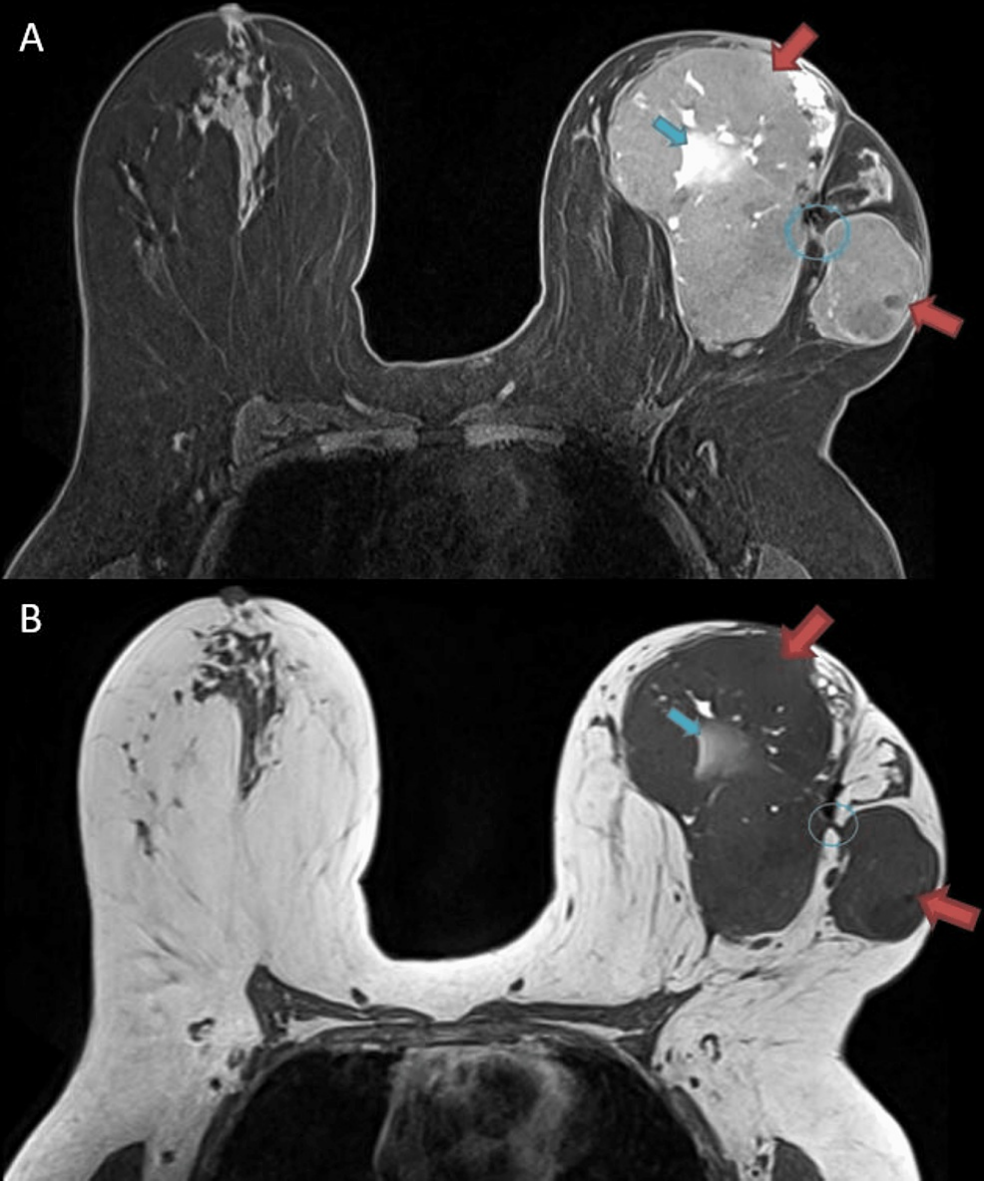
A) T2-weighted and B) T1-weighted MRI of the left breast was significant for two heterogeneously enhancing masses interconnected at the site of the prior surgical scar and clip representing a single process (red arrows; blue circle) The conglomeration measures 13.0 x 15.0 x 14.0 cm (AP × TR × CC). There is apparent, necrosis within the dominant portion of the lesion (blue arrow).
